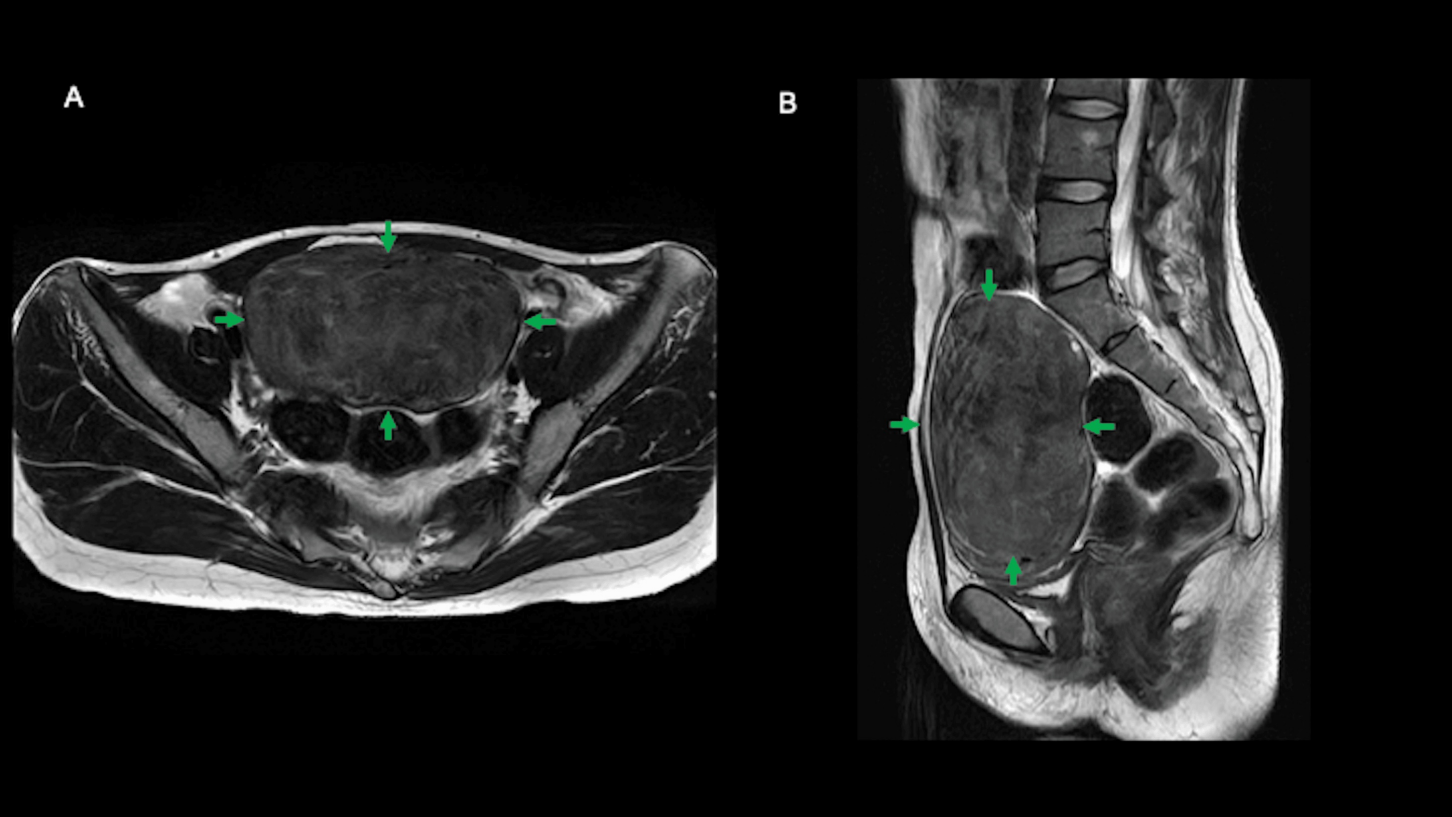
T2-weighted magnetic resonance imaging after preoperative hormone therapy with GnRH agonist (4 months), shown in the axial (A) and sagittal (B) views The pelvic mass (green arrow) showed a reduction in the maximum diameter to 12 cm.
